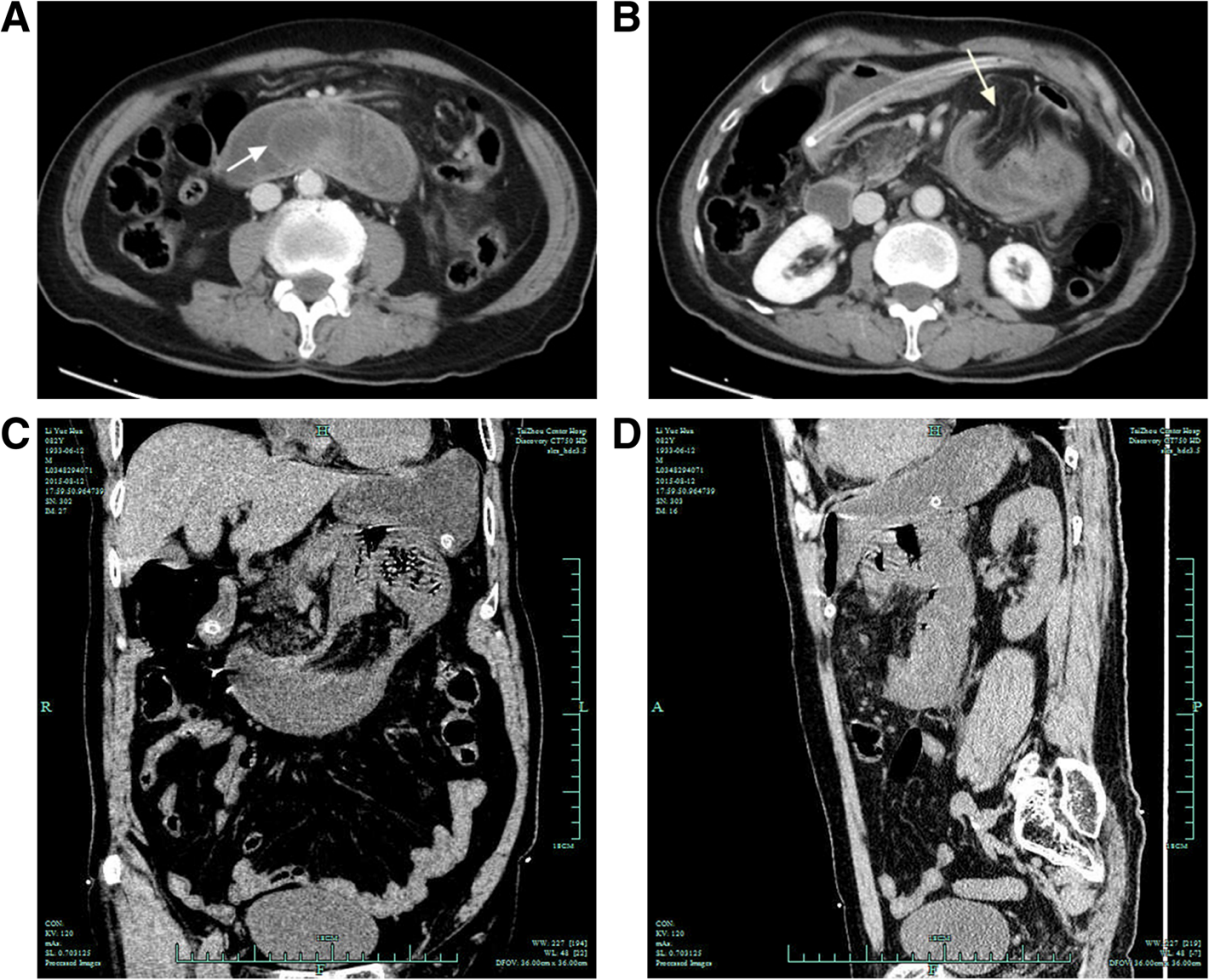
The patient showed typical target-ring signs (white arrow) with part of the jejunum enclosed with the horizontal segment of the duodenum (a). Aberrant condensation was noticed in the small intestine in the left upper abdomen. The upper segment enclosed with the jejunum paraduodenal recess, which was represented by sac structures (white arrow, b). c, d Coronal and sagittal findings of CT
